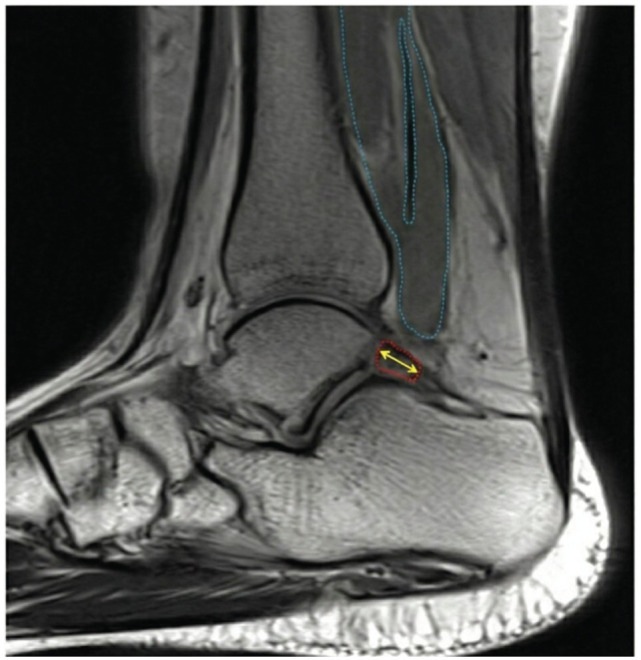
Mid-sagittal T1-weighted image shows a 38.9-mm^2^ OT area measurement (red outline) and the OT length measurement (yellow arrow) on the left ankle of a 16-year-old female with OT syndrome. The FHL muscle belly and tendon are also visualized and outlined in blue. FHL, flexor hallucis longus; OT, os trigonum.
